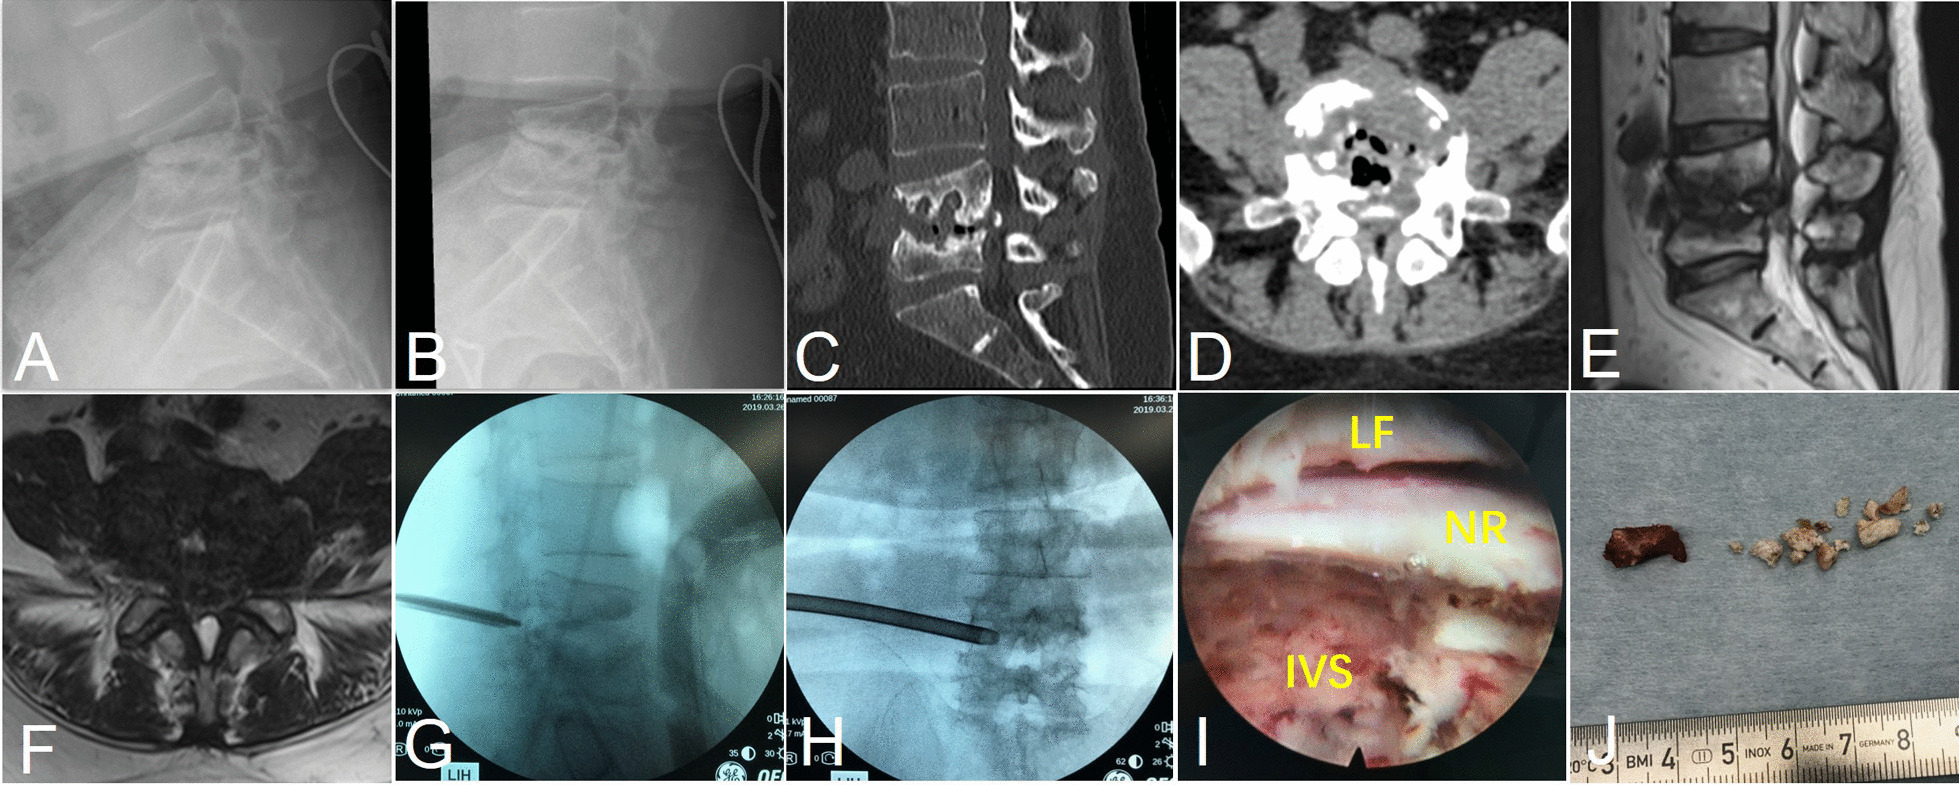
Images of the lesion before or at the second endoscopic surgery (foraminoplasty and discectomy). The dynamic roentgenography (A, B), CT (C, D), and MR T2WI (E, F) images of the second administration, intraoperative localization (G, H), and endoscopic view (I) after foraminoplasty and discectomy (J) were presented. *LF* ligamentum flavum, *NR* nerve root, *IVS* intervertebral space
